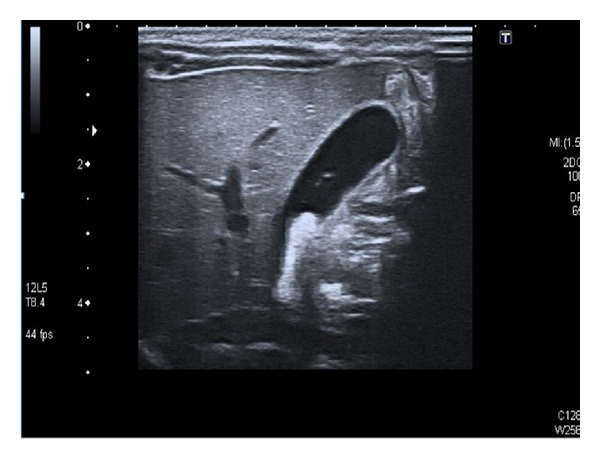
Case 2: neonatal ultrasound image showing fetal gallbladder sludge obstructing the bile duct.
